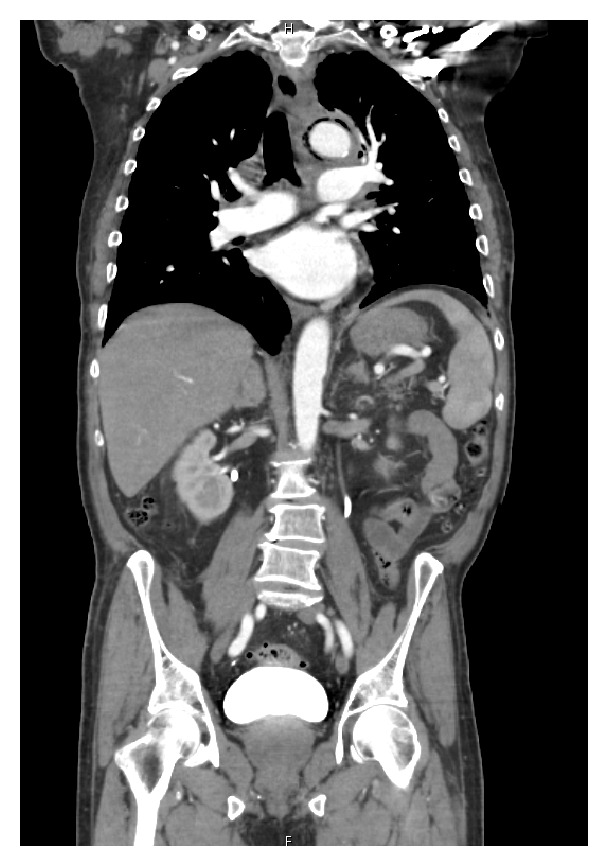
CT chest (coronal view) showing emphysematous aortitis.
